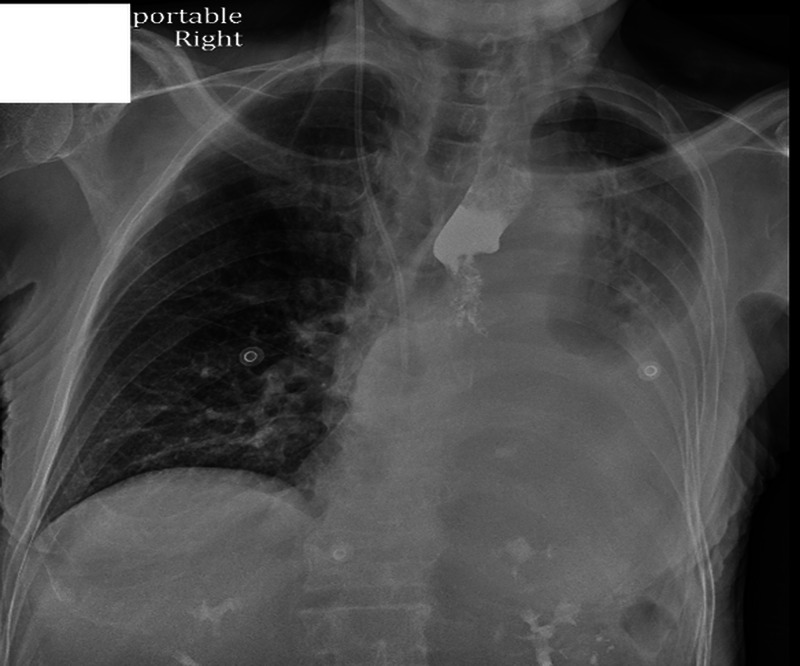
Subsequently, a chest x-ray showed persistently retained contrast within the esophagus and improved left lobar airspace disease with moderate to large pleural effusion.
